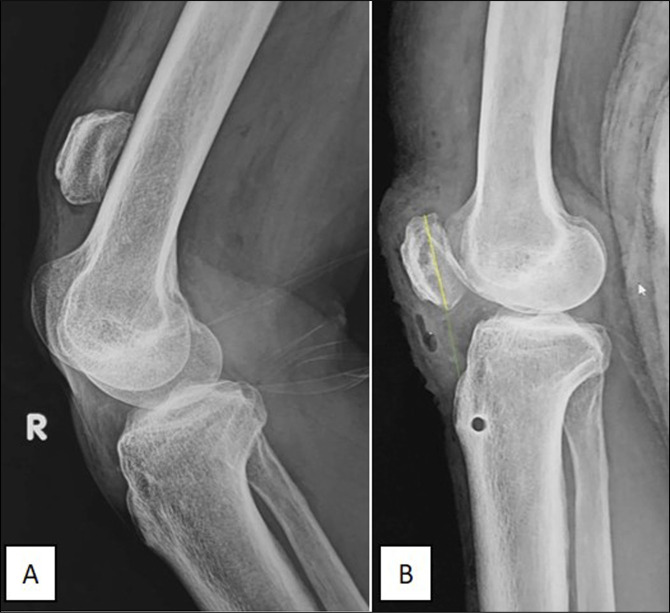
Plain radiographs of the right knee. A, Preoperative knee radiograph showing patella alta (B). Postoperative knee radiograph showing the patella in its anatomical position.
